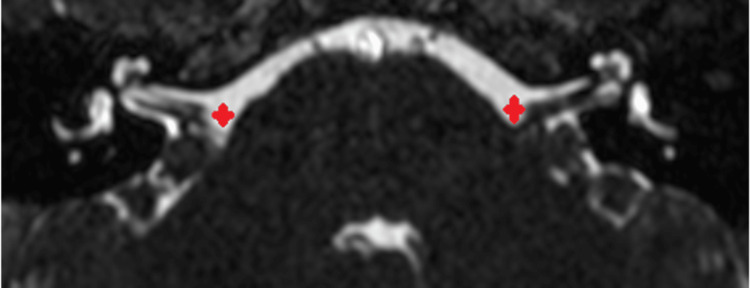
MRI with internal auditory canal protocol demonstrating normal anatomy of cranial nerves VIII and VII (marked in red).
